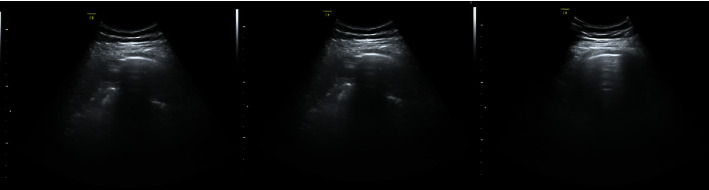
Two-dimensional ultrasound characteristics of patients with SPE (gestational age of 26 weeks).
